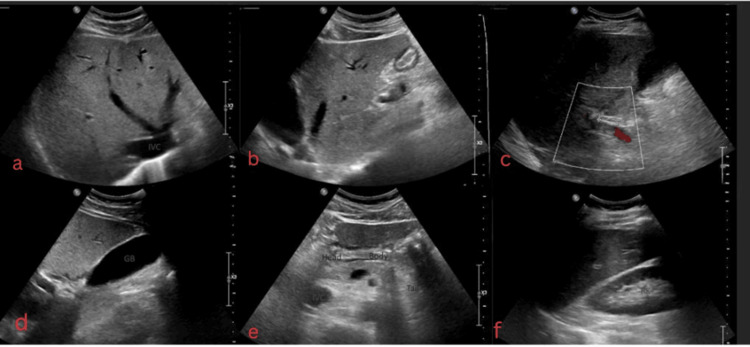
Transabdominal Ultrasound of the Abdomen (a) Transverse sonographic image of the right liver and (b) longitudinal ultrasound of the left hepatic lobe demonstrate normal homogeneous parenchyma with increased echogenicity, likely indicating underlying hepatocellular disease. (c) Common hepatic duct and common bile duct are not dilated (measured diameter: 0.464 cm). (d) Longitudinal gallbladder in supine position shows a normal-sized gallbladder; no gallstones are seen, and gallbladder wall thickness is normal. (e) The visualized portion of the pancreas is unremarkable, with normal parenchymal echotexture. (f) Longitudinal image of the right kidney demonstrates normal renal size, cortical thickness, and corticomedullary differentiation. No hydronephrosis, calculi, or focal masses are identified.
